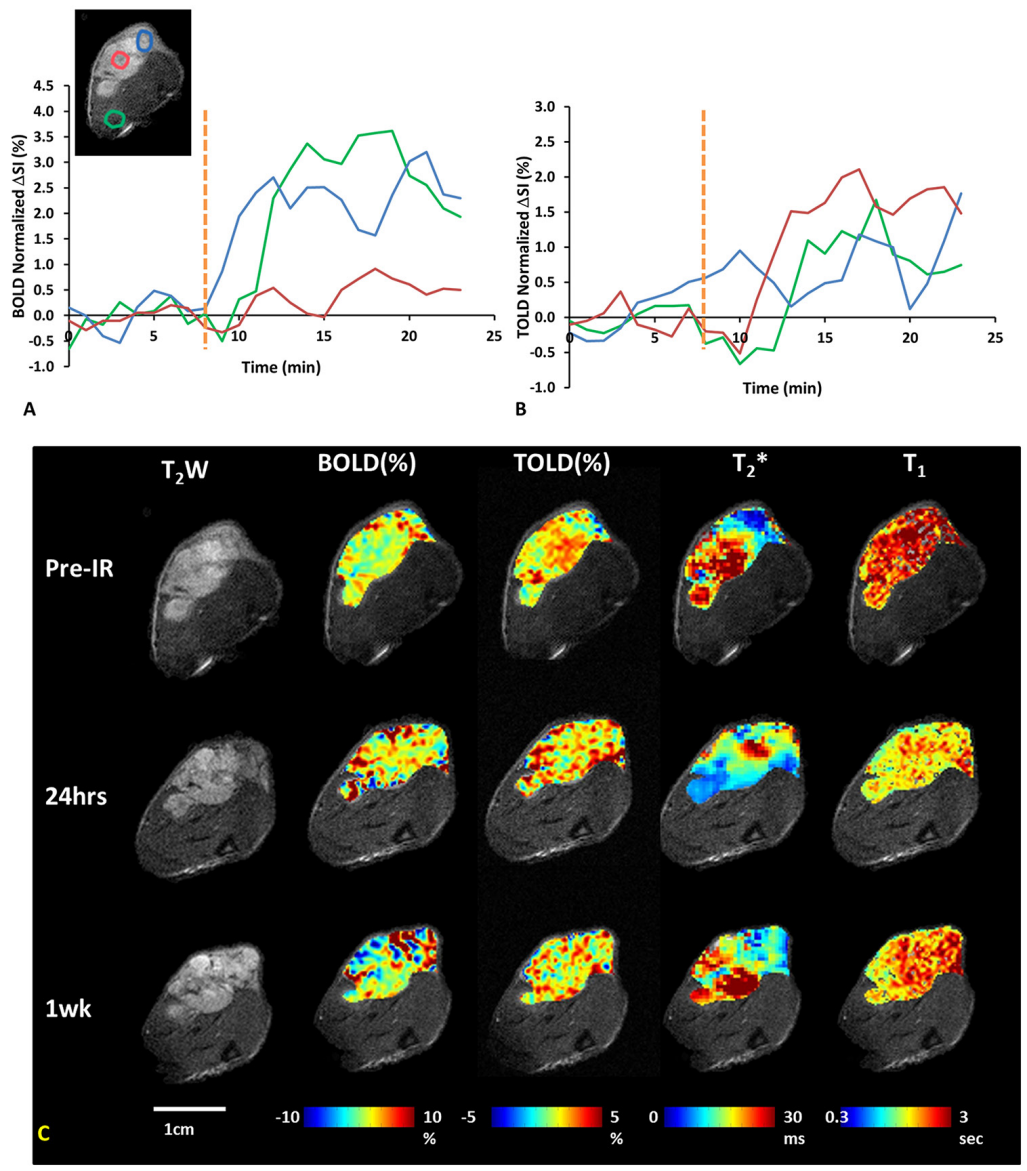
Oxygen sensitive MRI of a representative A549 lung tumor with respect to irradiation (A) ΔSI curves showing BOLD, and (B) TOLD responses for three ROIs indicated on T_2_w-image (blue tumor periphery, red tumor center and green adjacent muscle). Yellow dotted line indicates transition from air to oxygen breathing. (C) T_2_w-images and overlaid parametric maps showing %ΔSI of BOLD and TOLD, T_2_* and T_1_ maps (left to right) of tumor (0.5 cm^3^) before, 24 hours and one week after 1^st^ dose of radiation (12 Gy).
